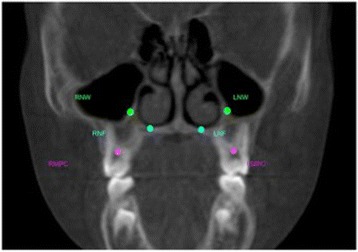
Dental and skeletal landmarks on a CBCT coronal scan. RMPC, right molar pulp chamber; LMPC, left molar pulp chamber; RNF, right nasal floor; LNF, left nasal floor; RNW, right nasal wall; LNW, left nasal wall
